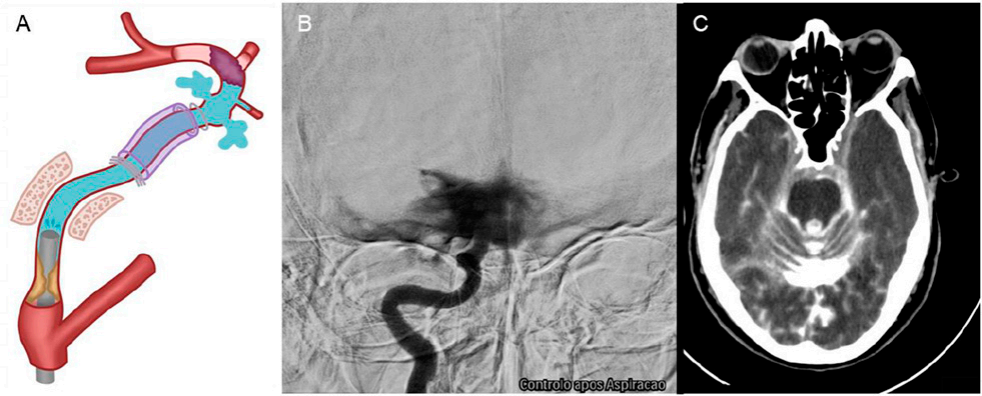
(A) Graphic representation of the internal carotid artery’s wall rupture beyond the distal dural ring. (B) Extensive contrast extravasation and rupture of the internal carotid artery. (C) CT scan with extensive subarachnoid haemorrhage.
